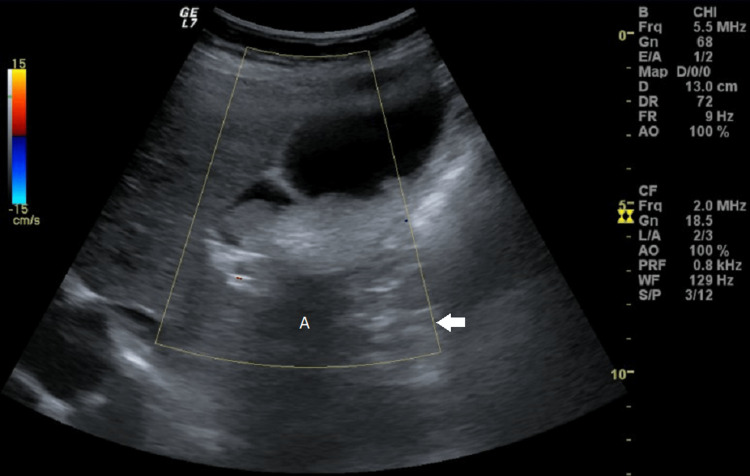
Ultrasound imaging of movable intraluminal echogenic mass-like gallbladder lesion, without posterior acoustic shadowing, internal vascularity absents by color Doppler ultrasound high attenuated. Arrow: Color Doppler field; A: Artifact resembling posterior acoustic shadowing but without typical features of gallstones
